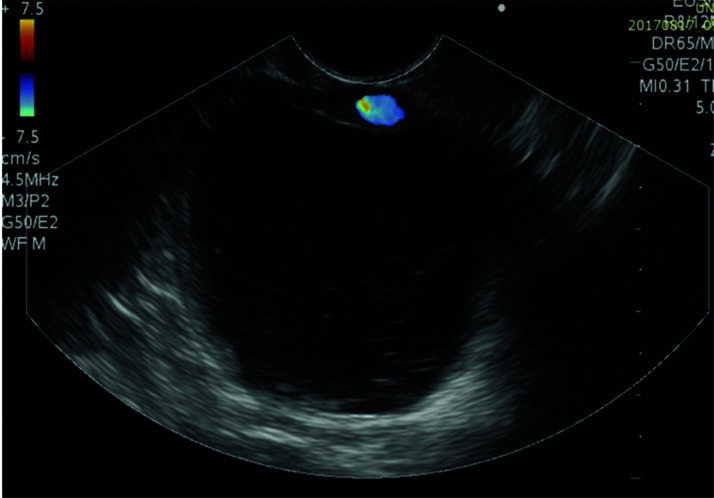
Identification of pancreatic pseudocysts on an endoscopic ultrasound image Doppler ultrasound was used to assess the gastric or duodenal wall for interposed vessels.
